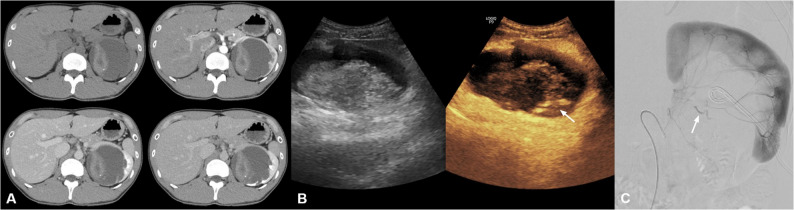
Renal arterial bleeding after blunt trauma. A Multiphase contrast-enhanced CT images (pre-contrast, arterial, portal venous, and delayed phases) demonstrate high-attenuation material within the left renal pelvis, consistent with hemorrhage, without definite active arterial extravasation; however, a subtle increase on delayed-phase images raises suspicion for ongoing bleeding. B CEUS demonstrates focal jet-like contrast extravasation into the left renal pelvis, suggestive of active bleeding (arrow). C Digital subtraction angiography demonstrates active arterial extravasation from a segmental renal arterial branch (arrow)
